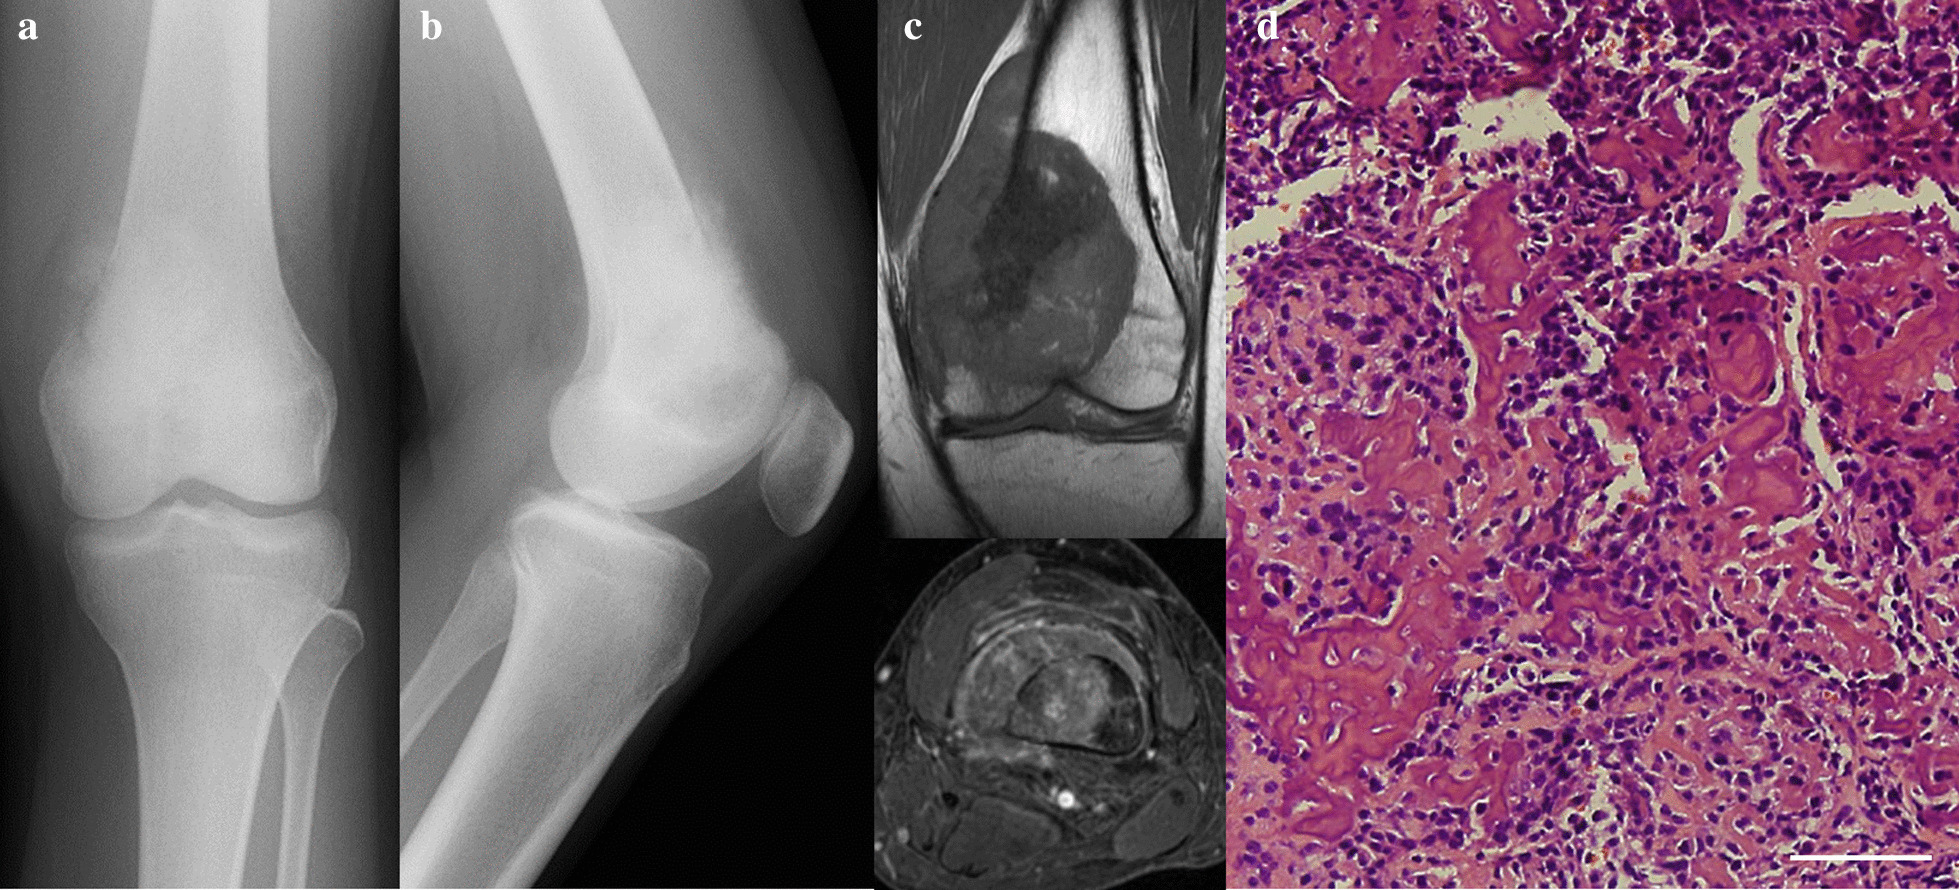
Radiological and pathological findings of a bone lesion at the left distal femur. a, b Preoperative roentgenogram on the anteroposterior view (a), and the lateral view (b). c Intraosseous lesion combined with extraosseous lesion on MRI was observed, which was hypointense signal on T1 weighted images, and hyperintense signal on T2 weighted images. d Enhanced MRI revealed unevenly contrasted lesion on axial images. e Proliferation of pleomorphic spindle cells with nuclear atypia and brisk mitosis, producing a large amount of lace-like osteoid, were observed. White scale bar shows 100 µm
